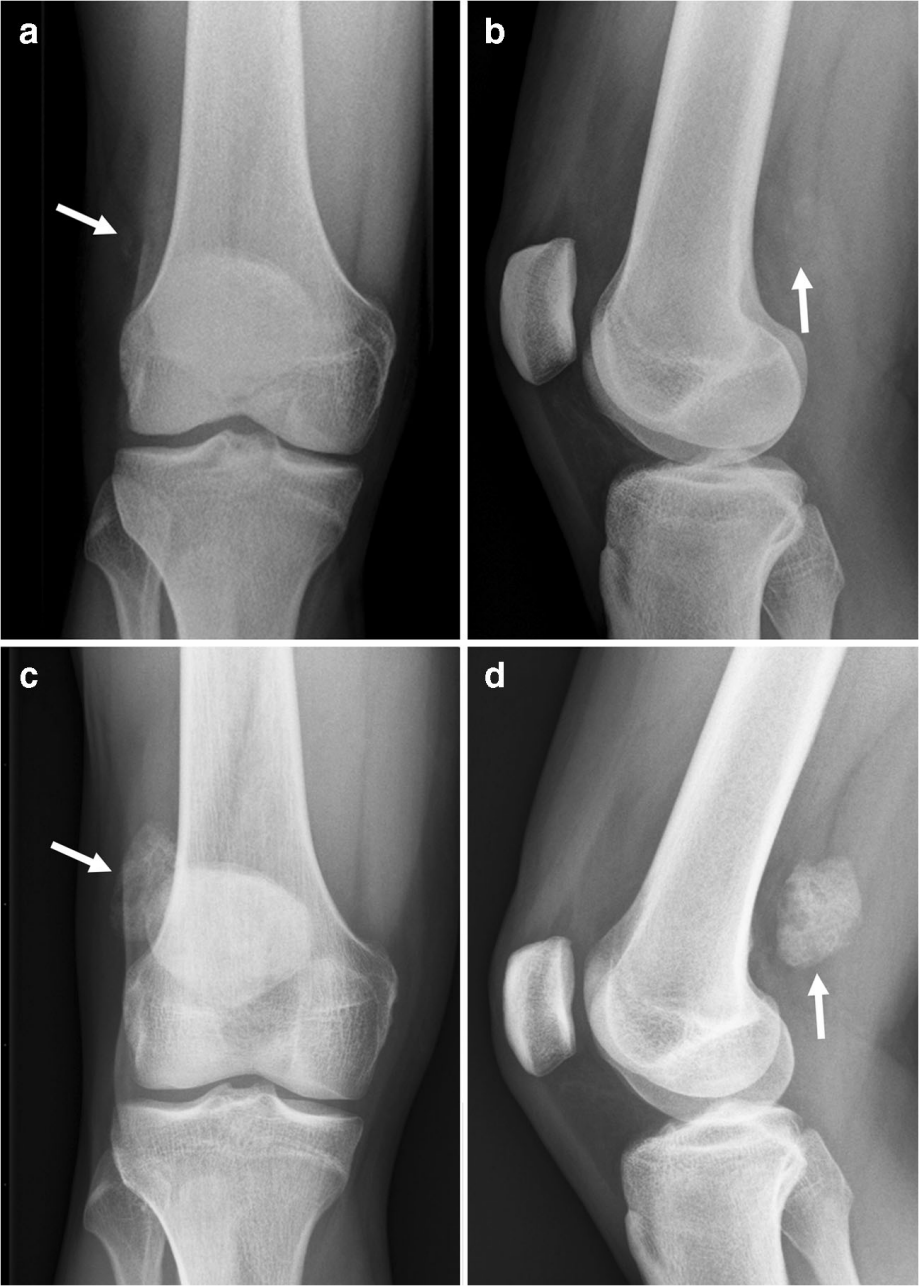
A 24-year-old man with myositis ossificans, early to intermediate stage (a, b) and late stage (c, d). a, b Initial radiography shows amorphous calcifications in the soft tissue at the posterolateral side of the distal femur. c, d Follow-up radiography after 1.5 years shows ossification
